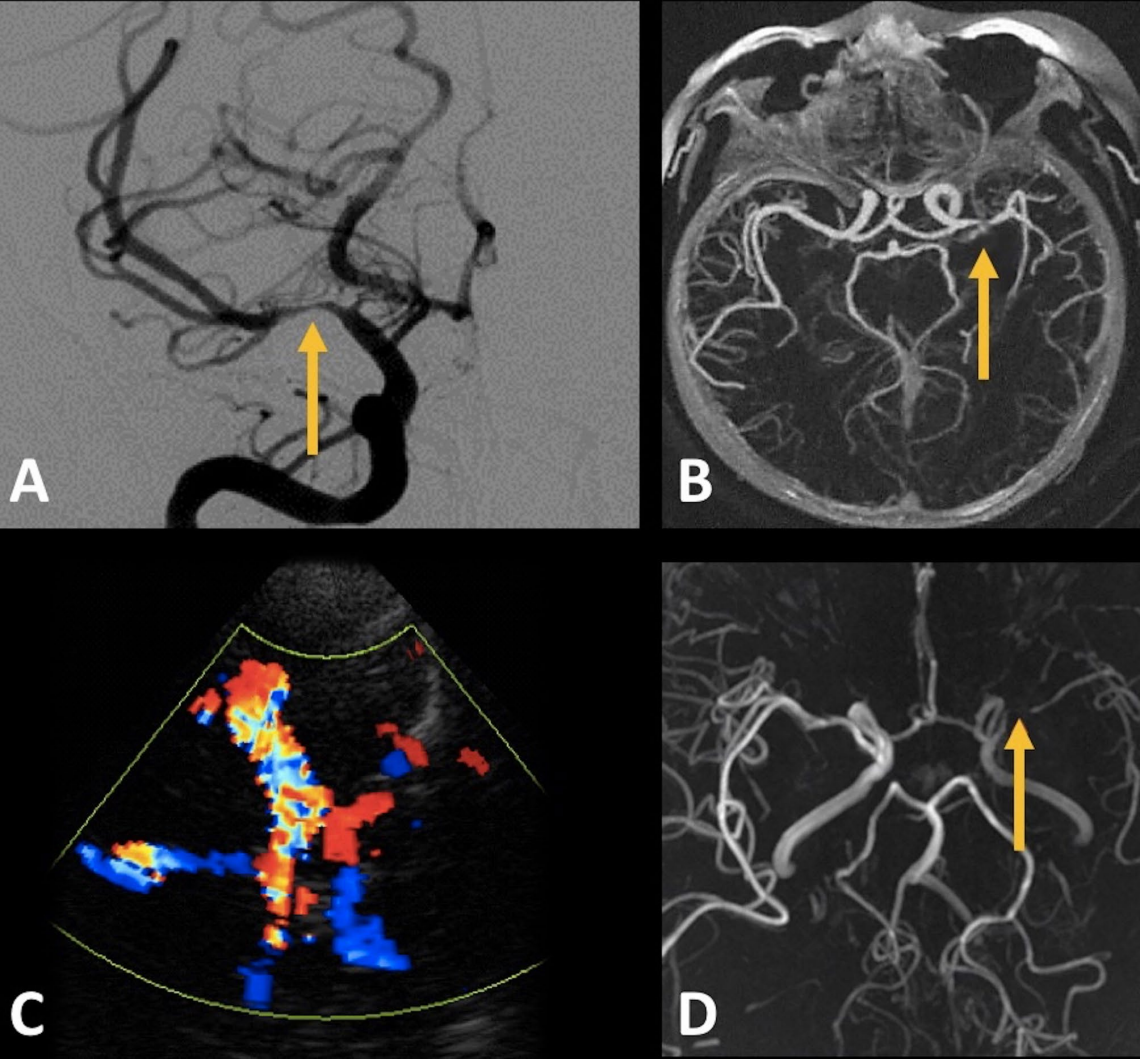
Diagnostic modalities for Intracranial Atherosclerosis. A: DSA reveals atherosclerotic stenosis of the M2 segment of the right MCA; B: Axial CTA shows high-grade stenosis of the M1 segment of the left MCA; C: Transcranial color-coded duplex ultrasonography (TCCS) reveals significant stenosis of the M1 segment of the right MCA with aliasing phenomenon; D: Axial MRA shows a high-grade stenosis of the M1 segment of the left MCA Abbreviations: DSA: Digital Subtraction Angiography; MCA: middle cerebral artery; CTA: Computed Tomography Angiography; MRA: Magnetic Resonance Angiography
